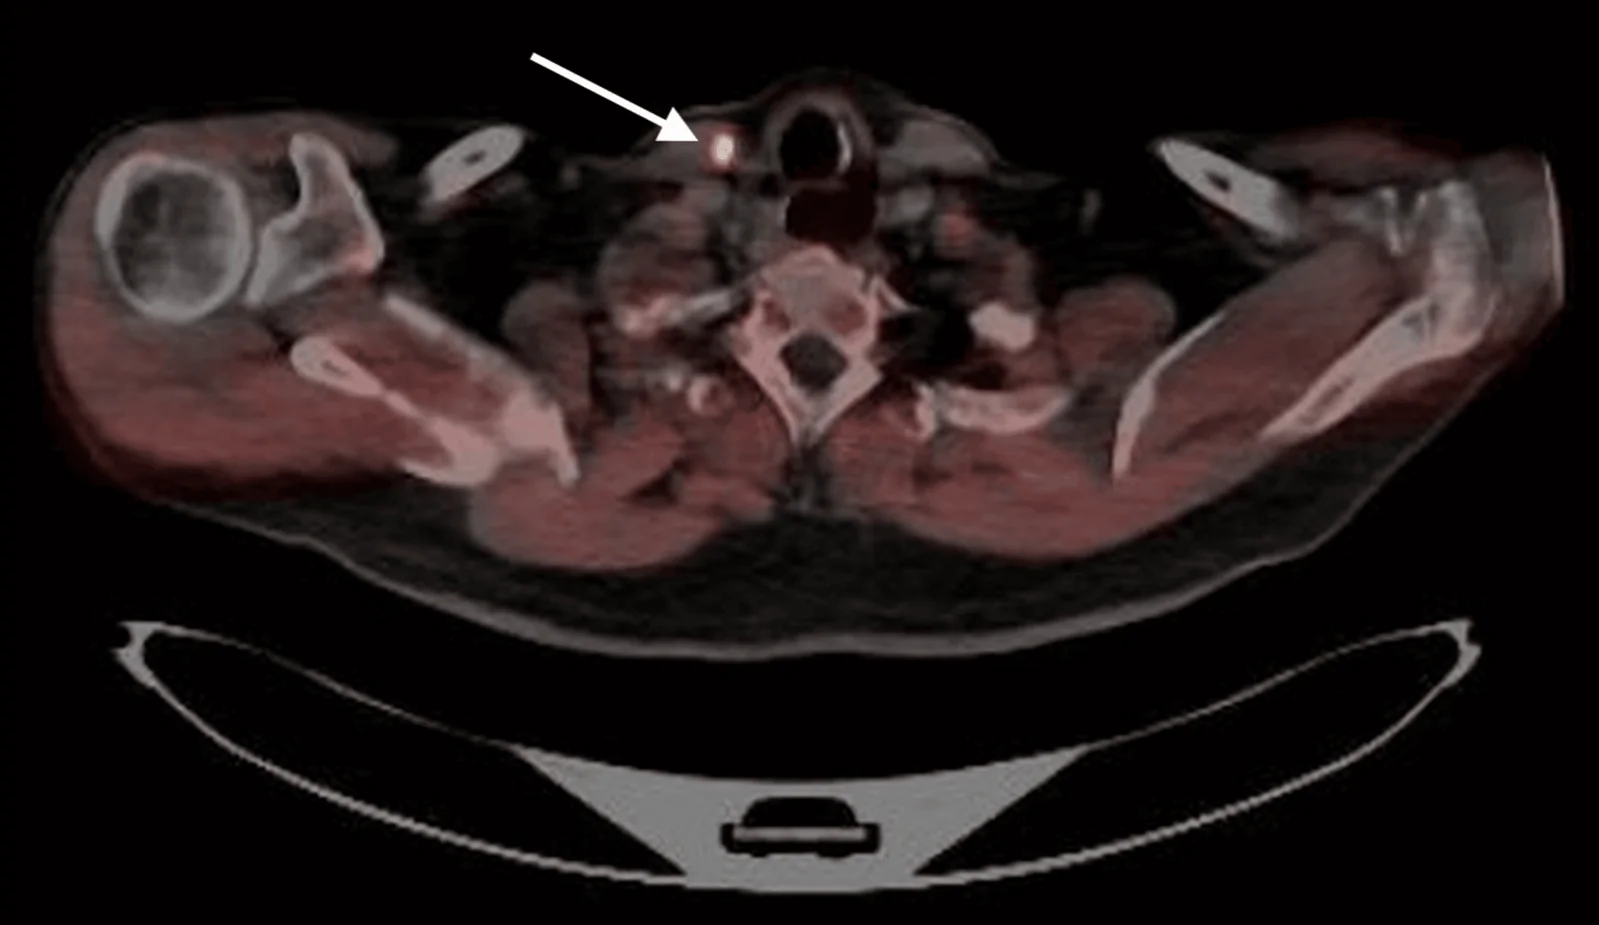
¹⁸F-choline PET/CT demonstrating focal tracer uptake within the autotransplanted parathyroid tissue. Axial ¹⁸F-choline PET/CT image demonstrating focal tracer uptake within the right sternocleidomastoid muscle at the site of previous parathyroid autotransplantation (arrow), consistent with a parathyroid adenoma arising within the autotransplanted parathyroid tissue.
